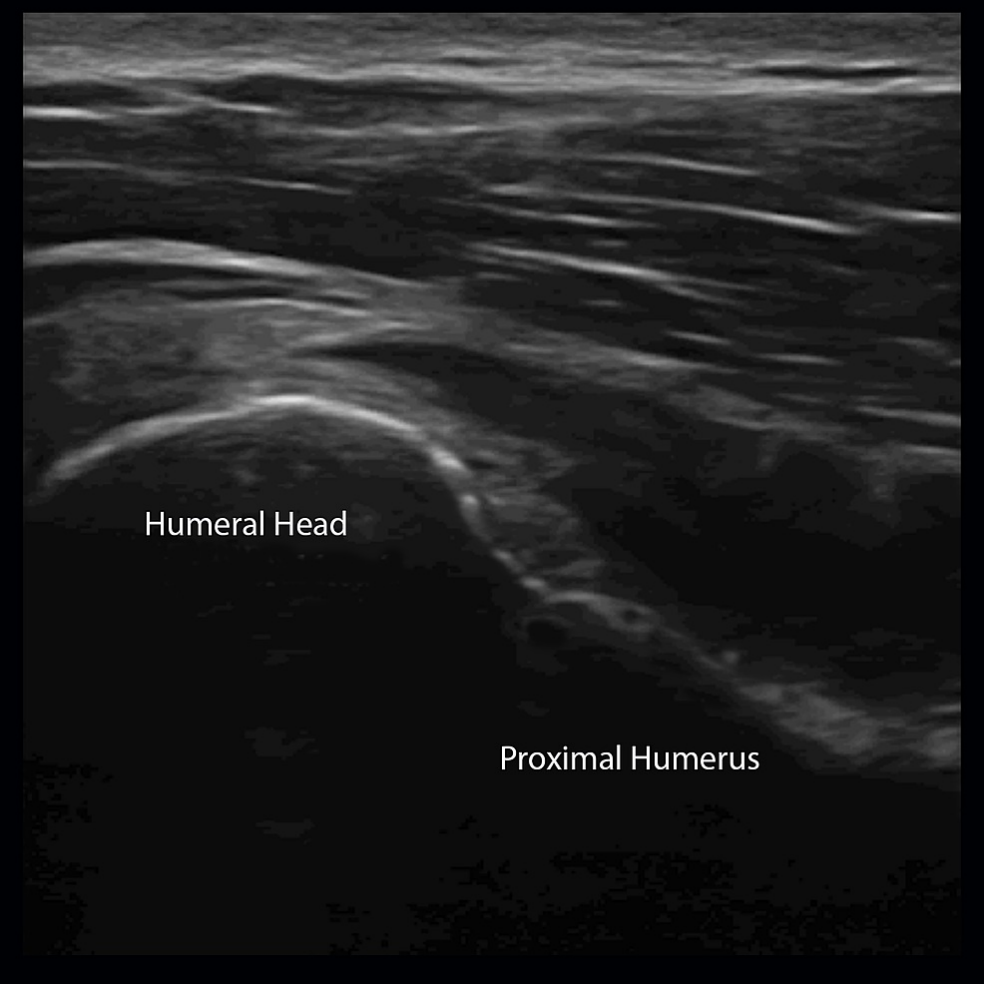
Proximal Humerus A coronal view of the humerus is obtained with the ultrasound probe with marker-oriented cephalad in order to visualize the humeral head and proximal humerus.
